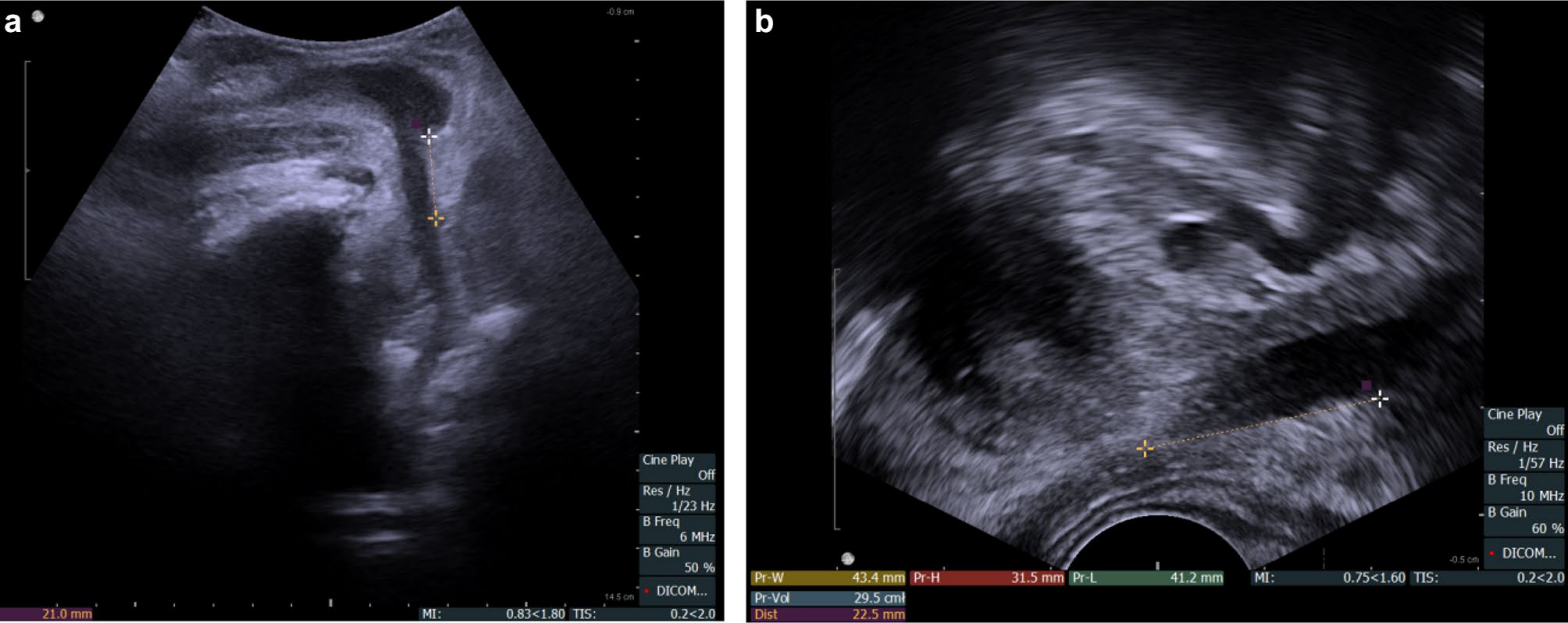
(a) Preoperative membranous urethra length—MULpre = 21 mm, measured in transperineal ultrasound—TPUS. (b) Preoperative membranous urethra length—MULpre = 22.5 mm, measured in transrectal ultrasound—TRUS.
